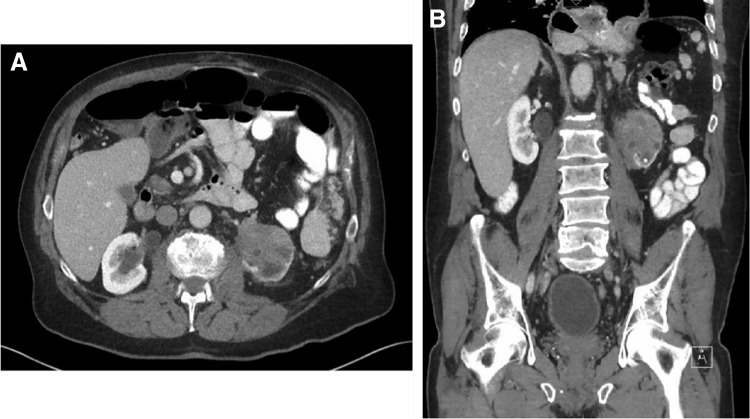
CT scan with axial (A) and coronal (B) views showing an infiltrating mass in an atrophic left kidney. CT, computed tomography.
